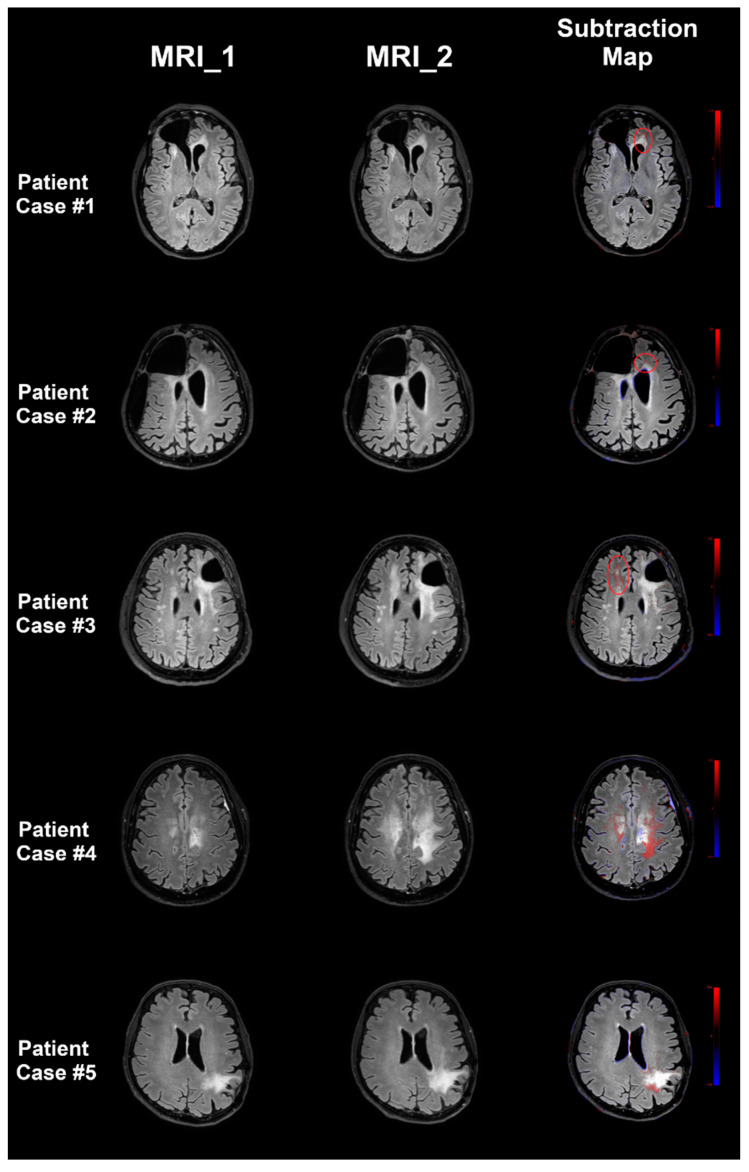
Exemplary patient cases. Five exemplary patient cases with increasing fluid attenuated inversion recovery (FLAIR) signal, thus suspected (pseudo)progression, are shown in this figure. Axial slices of the FLAIR sequences of MRI_1 and MRI_2 as well as the subtraction maps (SMs) are shown, which use color-coding to show signal changes over time (new or progressive lesions are shown in red). For patient cases #1, #2, and #3, progressive FLAIR signal is rather subtle and is enclosed by red circles (located adjacent to the left-hemispheric anterior horn of the lateral ventricle for patient case #1, in the left-hemispheric frontal white matter close to an enlarged left-sided lateral ventricle in patient case #2, and in the right-hemispheric frontal white matter close to the lateral ventricle in patient case #3). In patient cases #4 and #5, progressive FLAIR signal was more extensive, and thus also easier to detect without SMs when comparing MRI_1 and MRI_2 (located in the bihemispheric centrum semiovale for patient case #4 and in the left-hemispheric parietal white matter extending to the lateral ventricle in patient case #5). For patient cases #1, #2, and #3, the FLAIR signal was determined as stable/unchanged by one reader each during conventional (CONV) reading that only used MRI_1 and MRI_2, whereas it was categorized as progressive by both readers according to the SM reading. The FLAIR signal for patient cases #4 and #5 was rated as progressive by both readers during CONV and SM reading, respectively.
